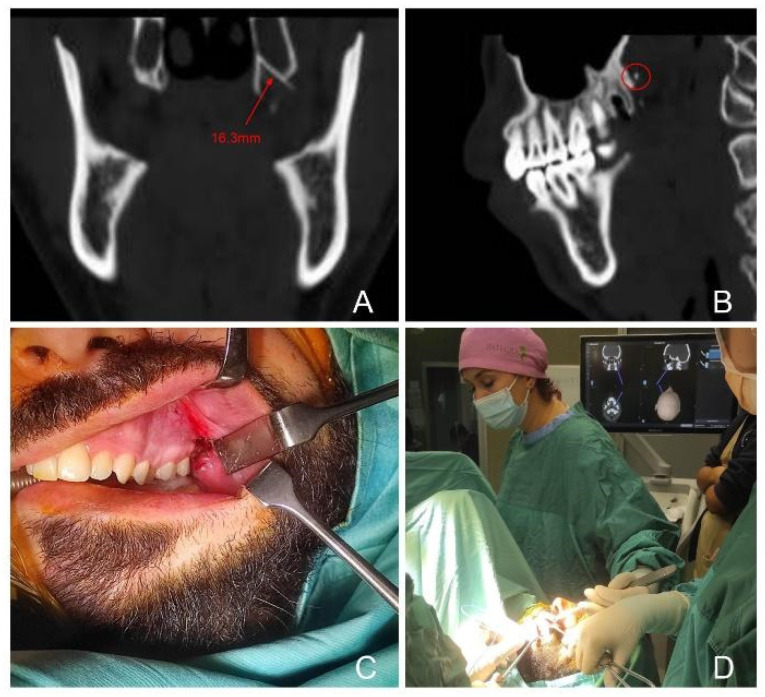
CT Coronal and sagittal scans locating the foreign body in the left pterygopalatine space (A,B); intraoperative removal from transoral approach and using CT-based intraoperative navigation (C,D). The foreign body is indicated by a dotted circle.
